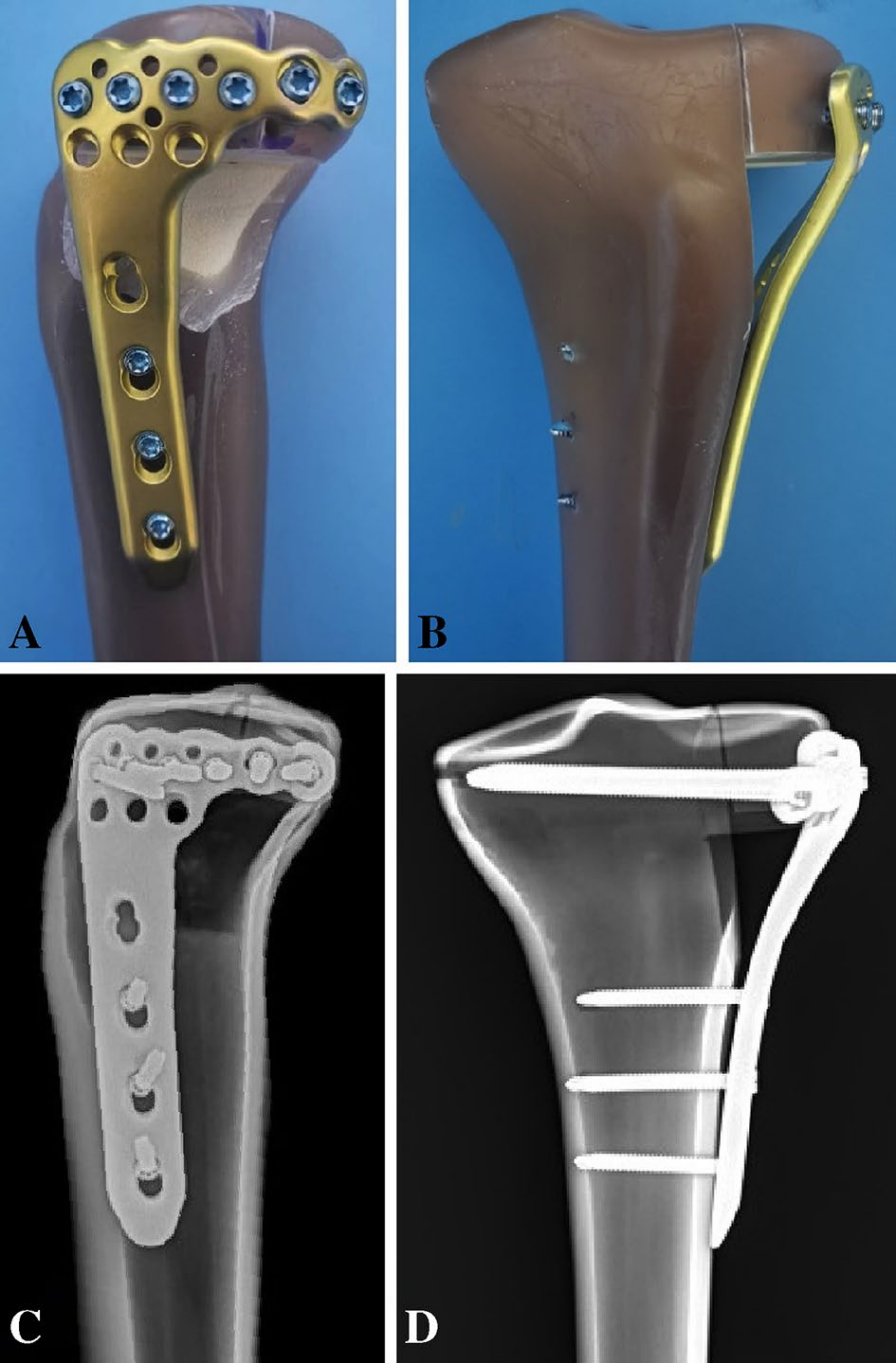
Mock-ups of the newly designed plate in synthetic bones. (A) Lateral view of the newly designed plate. (B) Anteroposterior view of the newly designed plate. (C) Lateral view of the X-ray image. (D) Anteroposterior view of the X-ray image.
